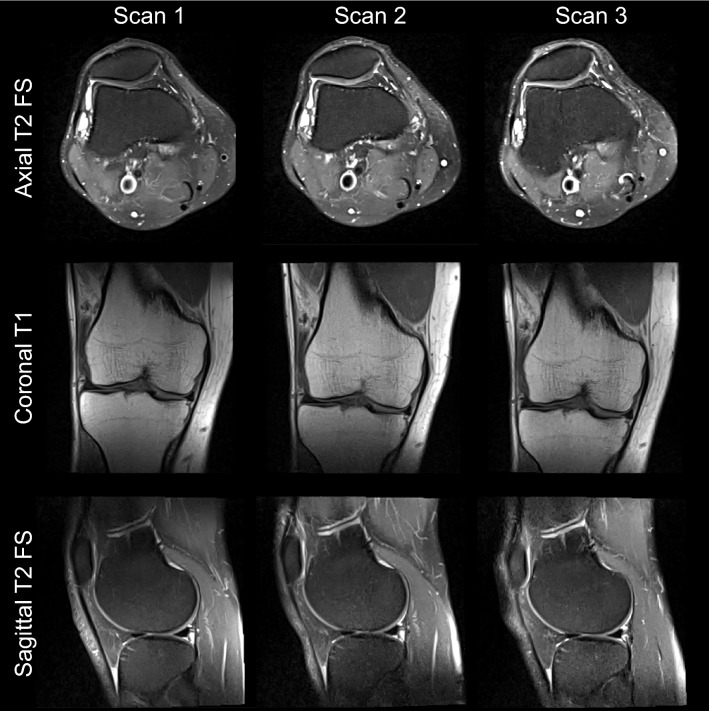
Turbo spin echo MR images from a 31-year-old man acquired with the 0.55 T cable coil during three separate scan sessions on the same day.
